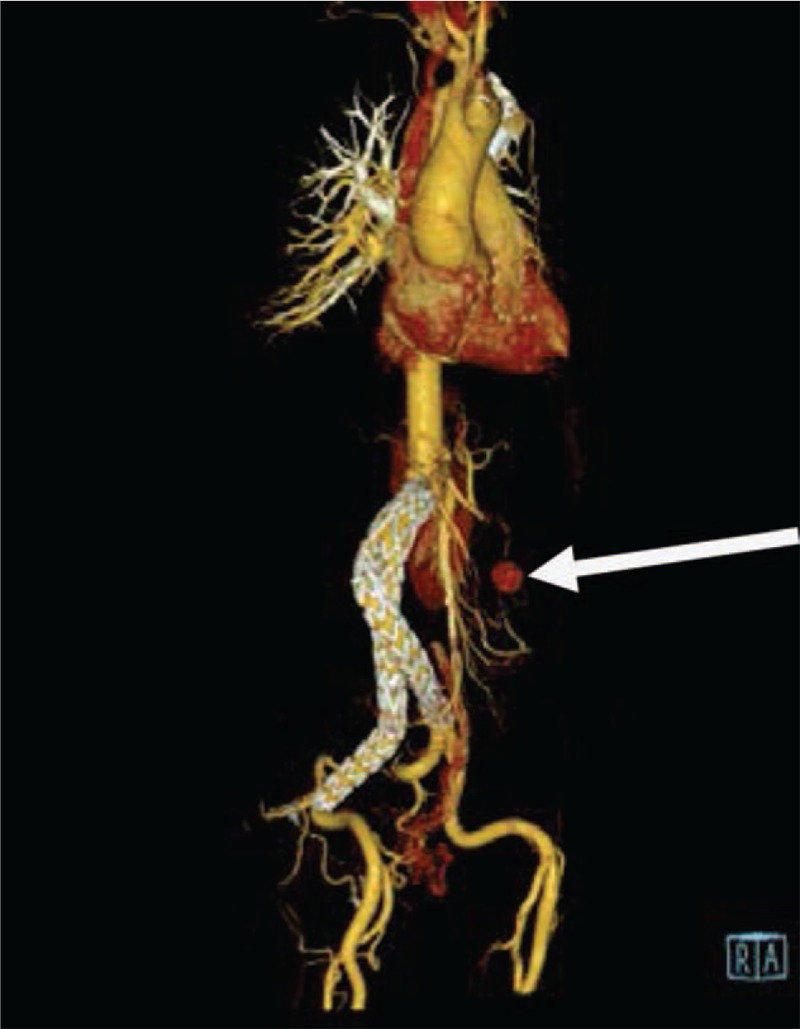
Preoperative aortic computed tomographic angiography (CTA) showing pseudoaneurysm signs in the distal end of a branch artery of superior mesenteric artery (SMA).
